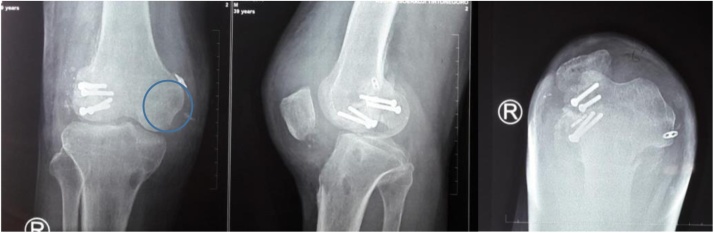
Anteroposterior, Lateral and Skyline view plain radiograph showed the lateral patellar dislocation.
